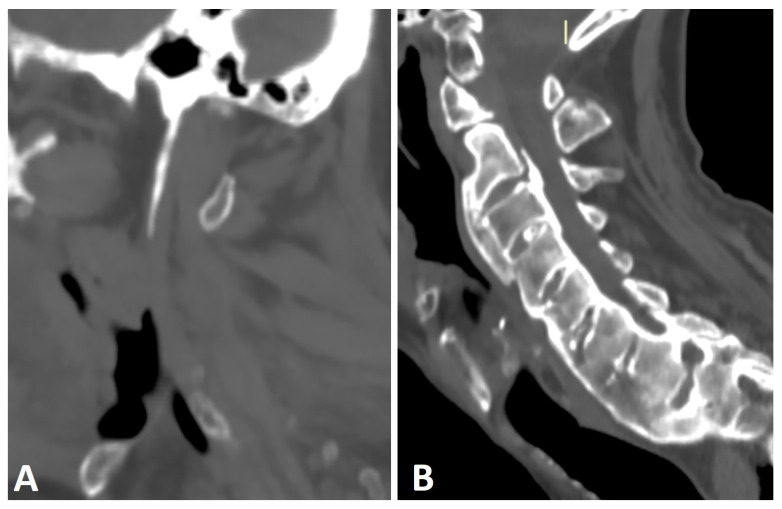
Sagittal CT reconstructions of the cervical spine of two patients with thoracic DISH (not shown). (A) The elongated styloid process results from calcification and ossification of the stylohyoid ligament. (B) Flowing osteophytes characteristic of DISH accompanied by OPLL.
